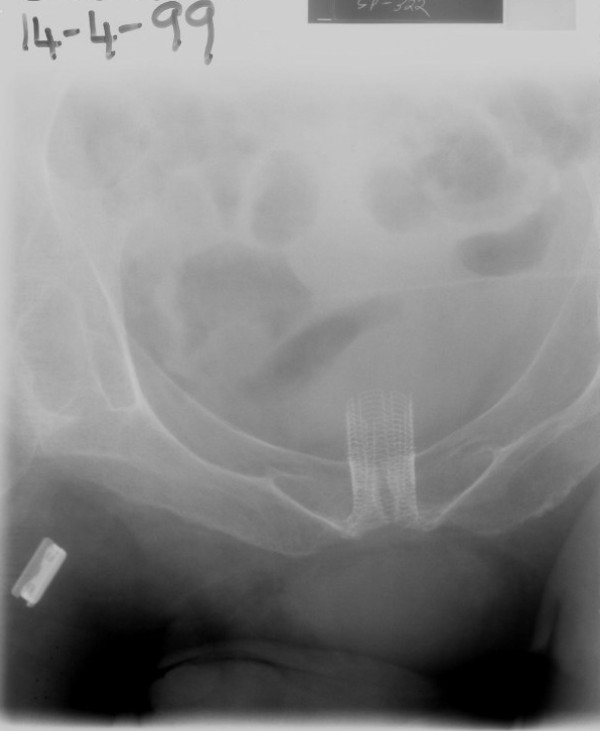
X-ray of urinary bladder (14 April 1999) shows UroLume stent. Urocoil stent had been removed.
